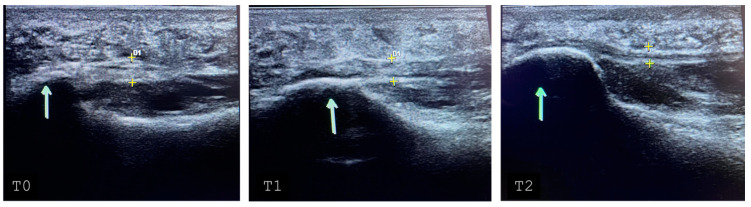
Ultrasound of the plantar fascia of a patient suffering from plantar fasciitis belonging to the laser group. At T0, there was a band thickness of 5 mm, which reduced to 4 mm at T1 and T2 to 3 mm. The arrows point to the heel; + signs indicate plantar fascia thickness; the D1 (1st distance) indicates measurement.
